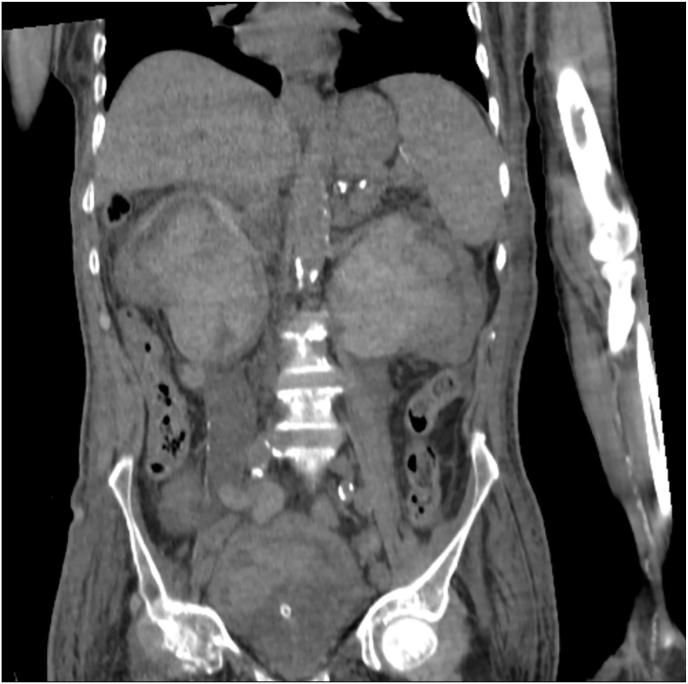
Non-contrast CT scan prior to fibrinolytic therapy, showing extensive clot burden filling the bilateral renal pelves and ureters, leading to significant hydronephrosis. The image highlights the challenging nature of the clot obstruction, extending beyond the bladder. Fig. 1 Non-contrast CT scan of the abdomen and pelvis of the patient prior to the administration of fibrinolytics.
